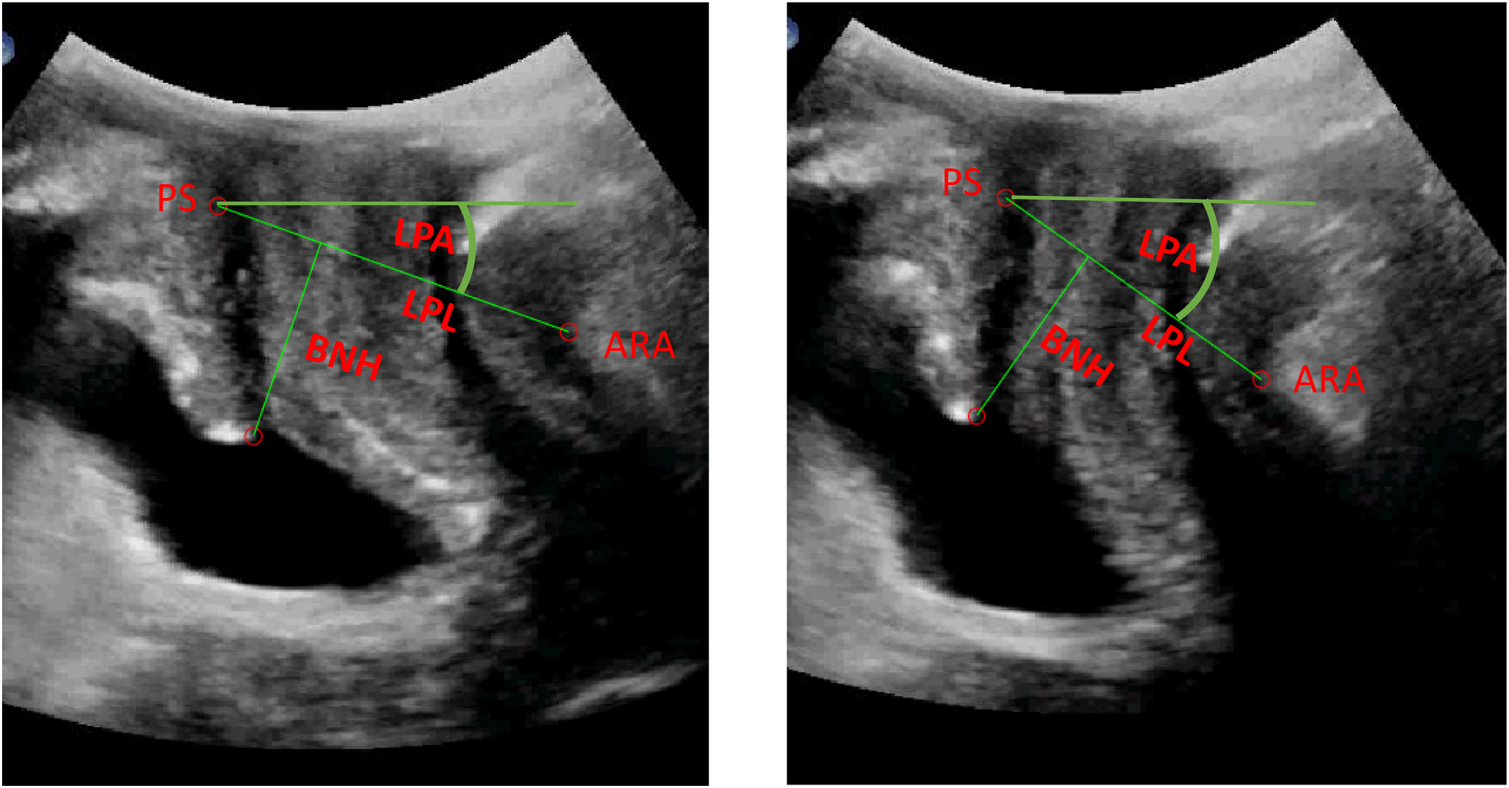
Transperineal ultrasound measure of pelvic muscle morphometry during rest (left) and max voluntary contraction (right): Bladder neck height (BNH), Levator plate length (LPL), and Levator plate angle (LPA), Pubic Symphysis (PS) and Anorectal Angle (ARA) are labeled on the images.
